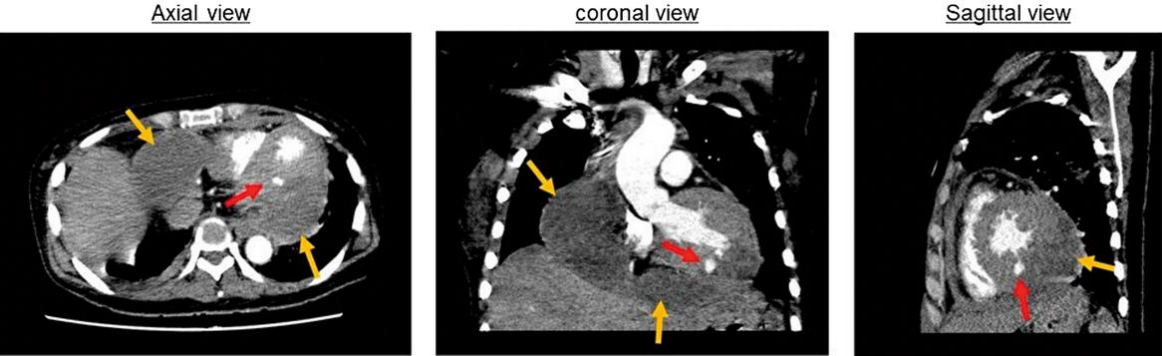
Contrast-enhanced computed tomography images of the chest. Contrast-enhanced computed tomography of the chest shows a large pericardial effusion of moderate density (orange arrows) and pseudoaneurysm on the inferior left ventricular wall (red arrows).
